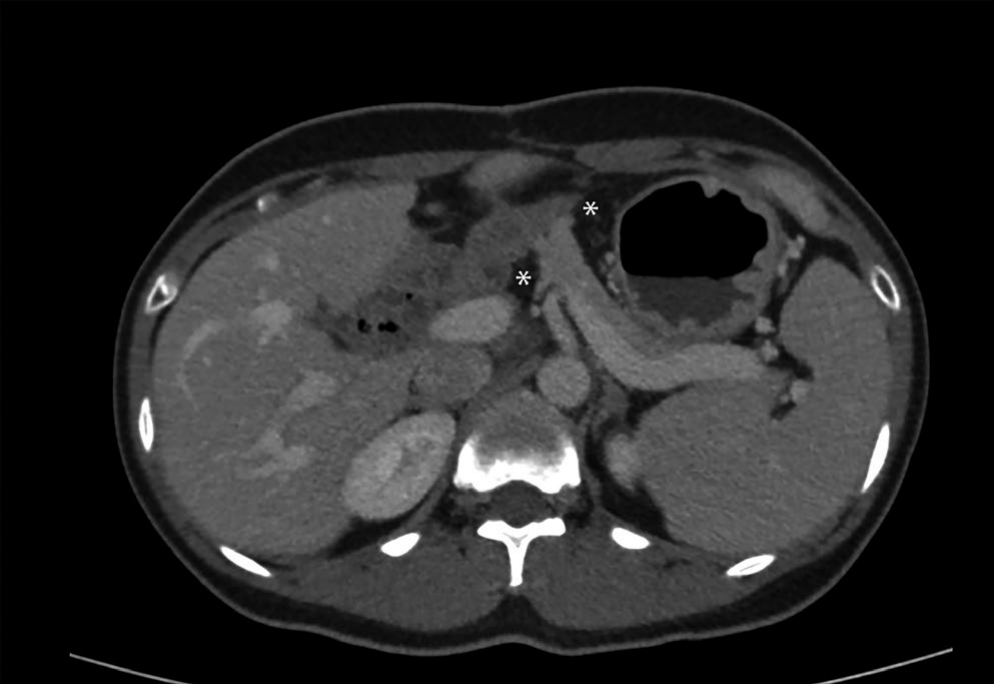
Computed tomography scan shows omentum around pancreatojejunal anastomosis without portal vein compression. *Omentum
